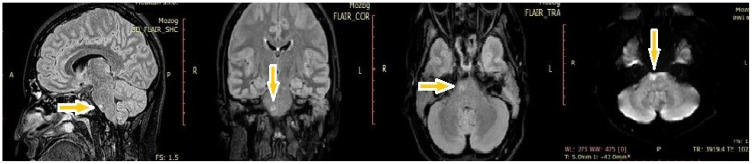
MRI findings of hyperintensities in the brainstem (yellow arrows) in FLAIR-weighted images and DWI.
